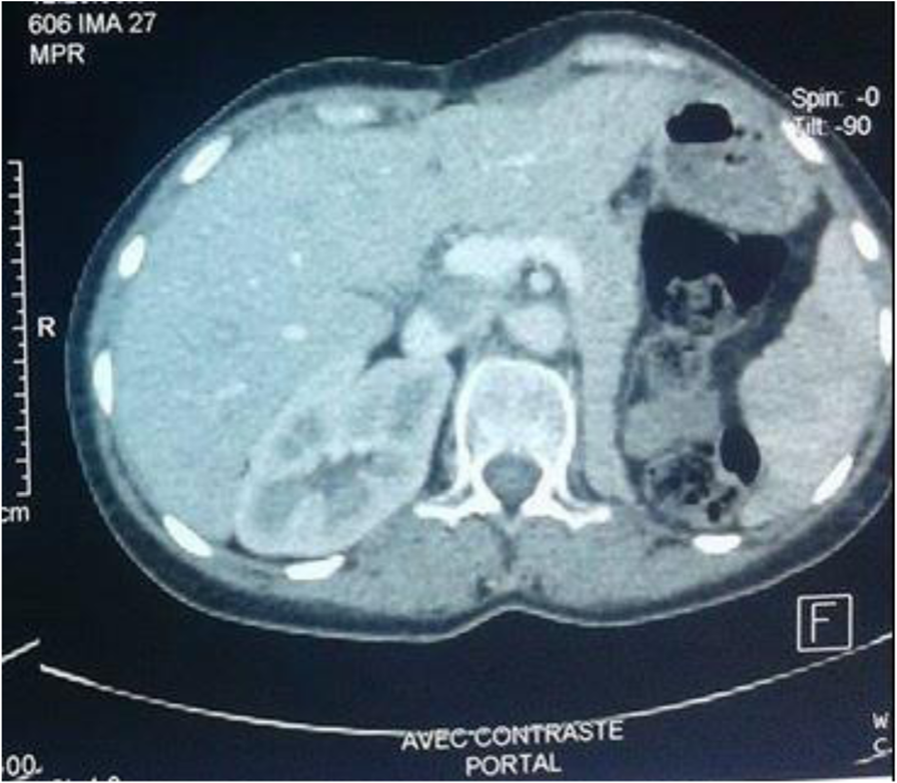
Post-chemotherapy axial computed tomography showing a partial response in the primitive site estimated to be 62%
